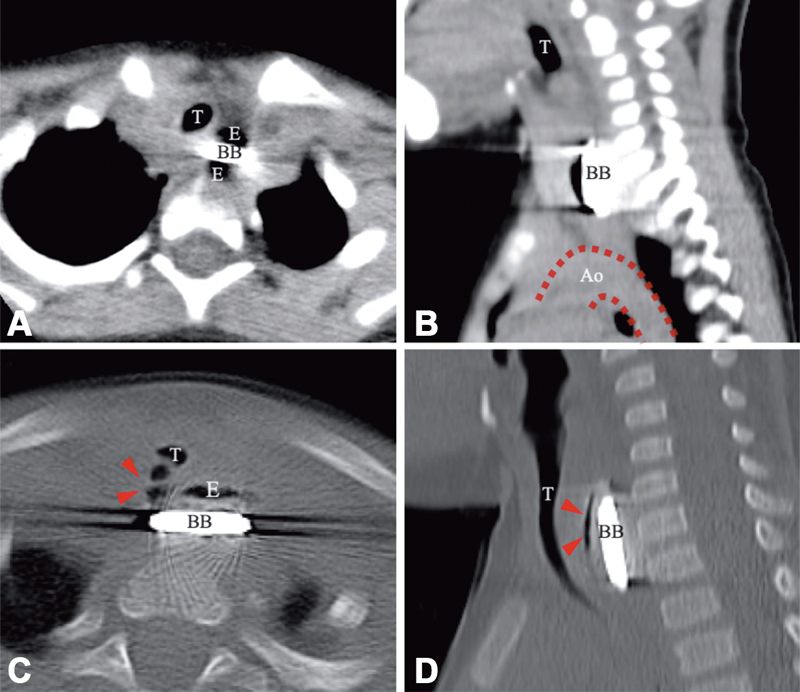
Computed tomography showing focal air in the mediastinum suggesting esophageal perforation. (
